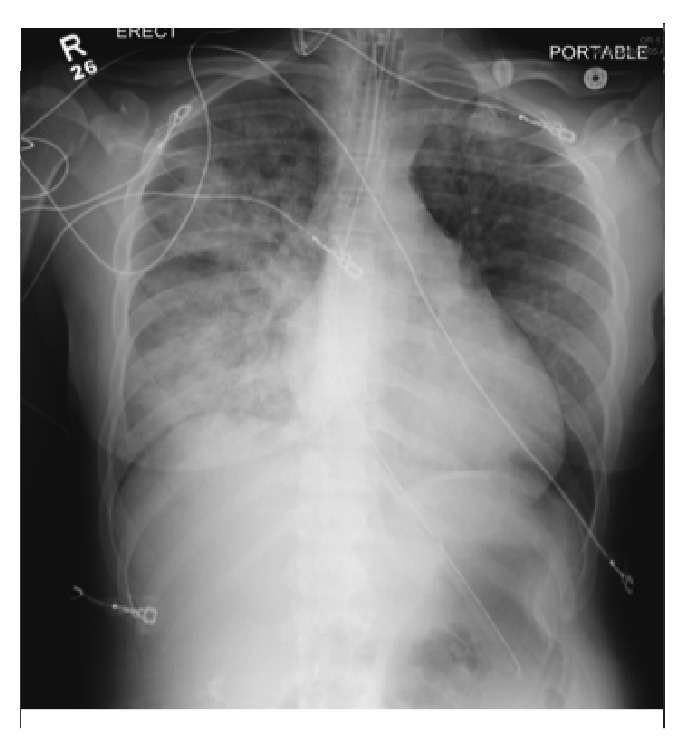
Chest X-ray: pulmonary edema with predominant right side opacities.
